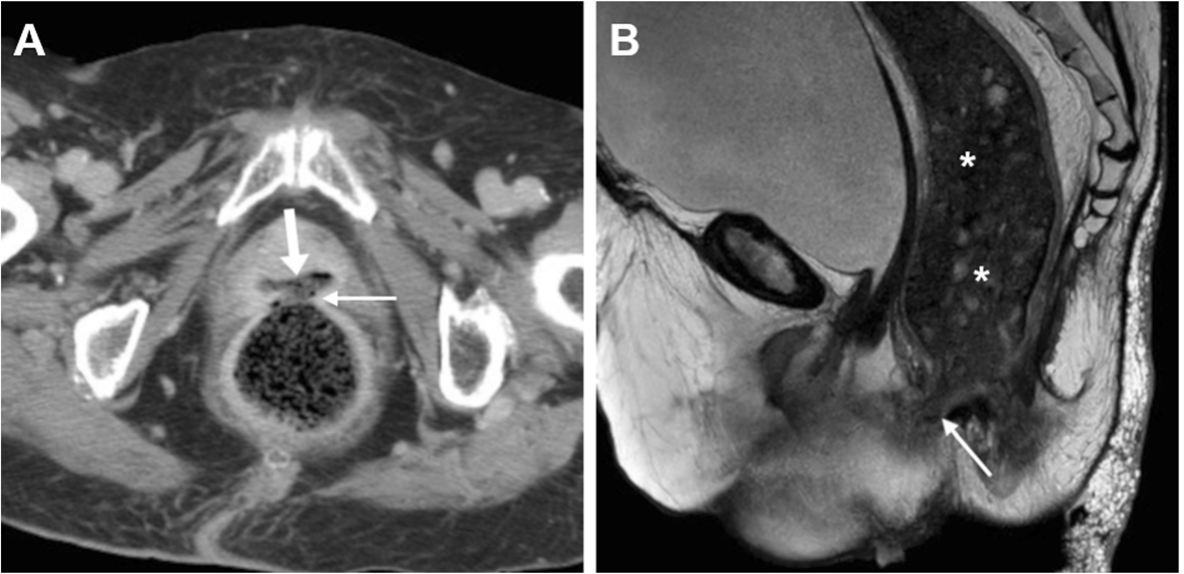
Two different radiation-induced recto-VF in elderly women with history of pelvic radiotherapy. a Axial CT image shows midline fistula (thin arrow) filled by faeces connecting the anterior aspect of the rectum to the vagina (arrow). b Sagittal T2-weighted MRI image shows a 1-cm wide fistula (thin arrows) between the most distal aspect of stool-filled rectum (*) and vagina
